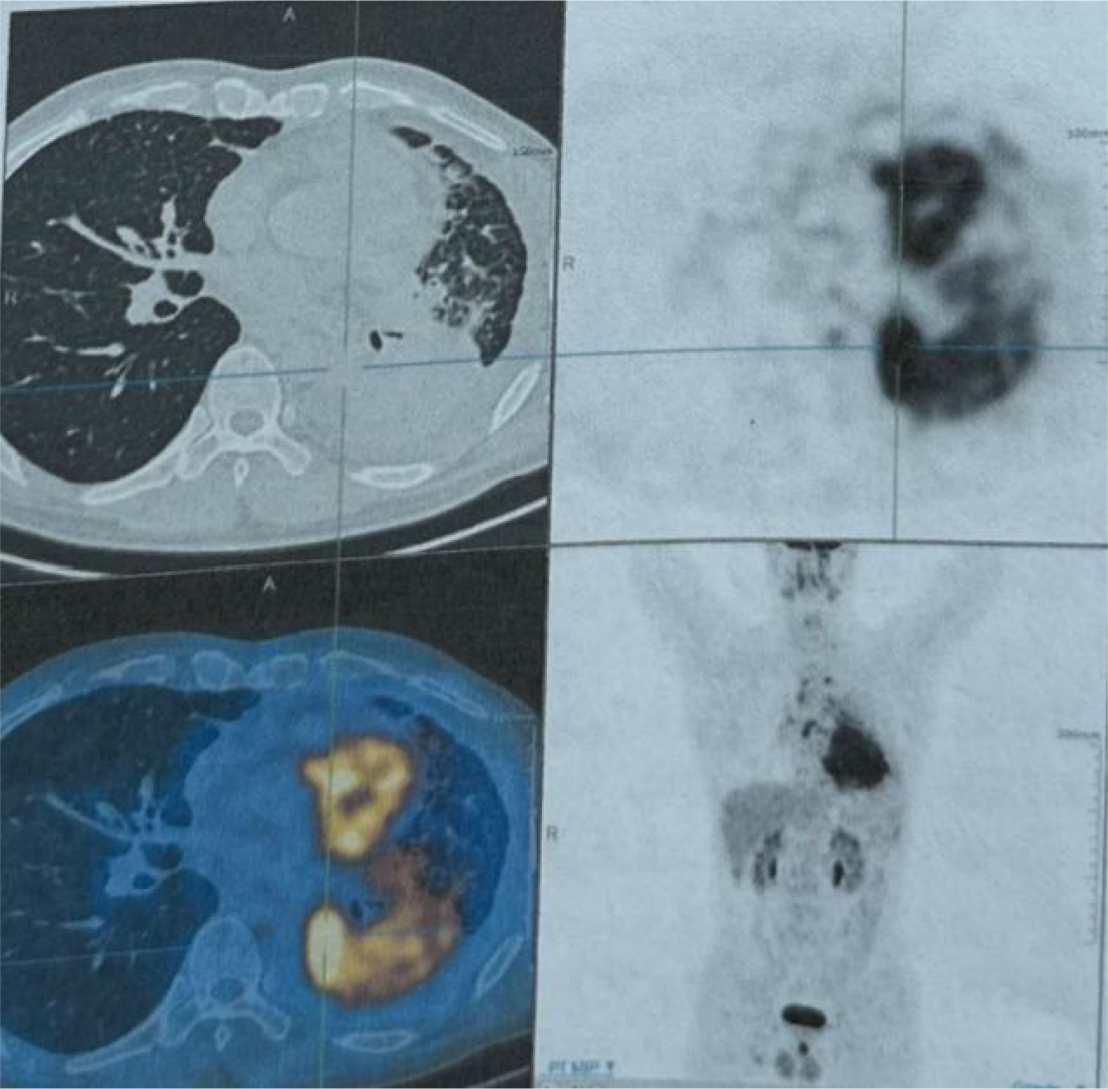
The PET-CT scan conducted on November 24, 2022, revealed a hypermetabolic mass in the left lower lobe of the lung, with a maximum SUV of 12.05 and a maximum transverse diameter measuring approximately 81.0 × 67.6 mm. The mass is indicative of central type lung cancer, accompanied by collapse of the left lung lower lobe and distal obstructive pneumonia. Additionally, there is evidence of lymph node metastasis in the left supraclavicular fossa, mediastinum, left pulmonary hilum, hepatogastric space, and retroperitoneum. Cancerous lymphangitis is observed in the left upper lobe, right middle lobe, and lower lobe, along with left-sided pleural effusion and pericardial effusion.
